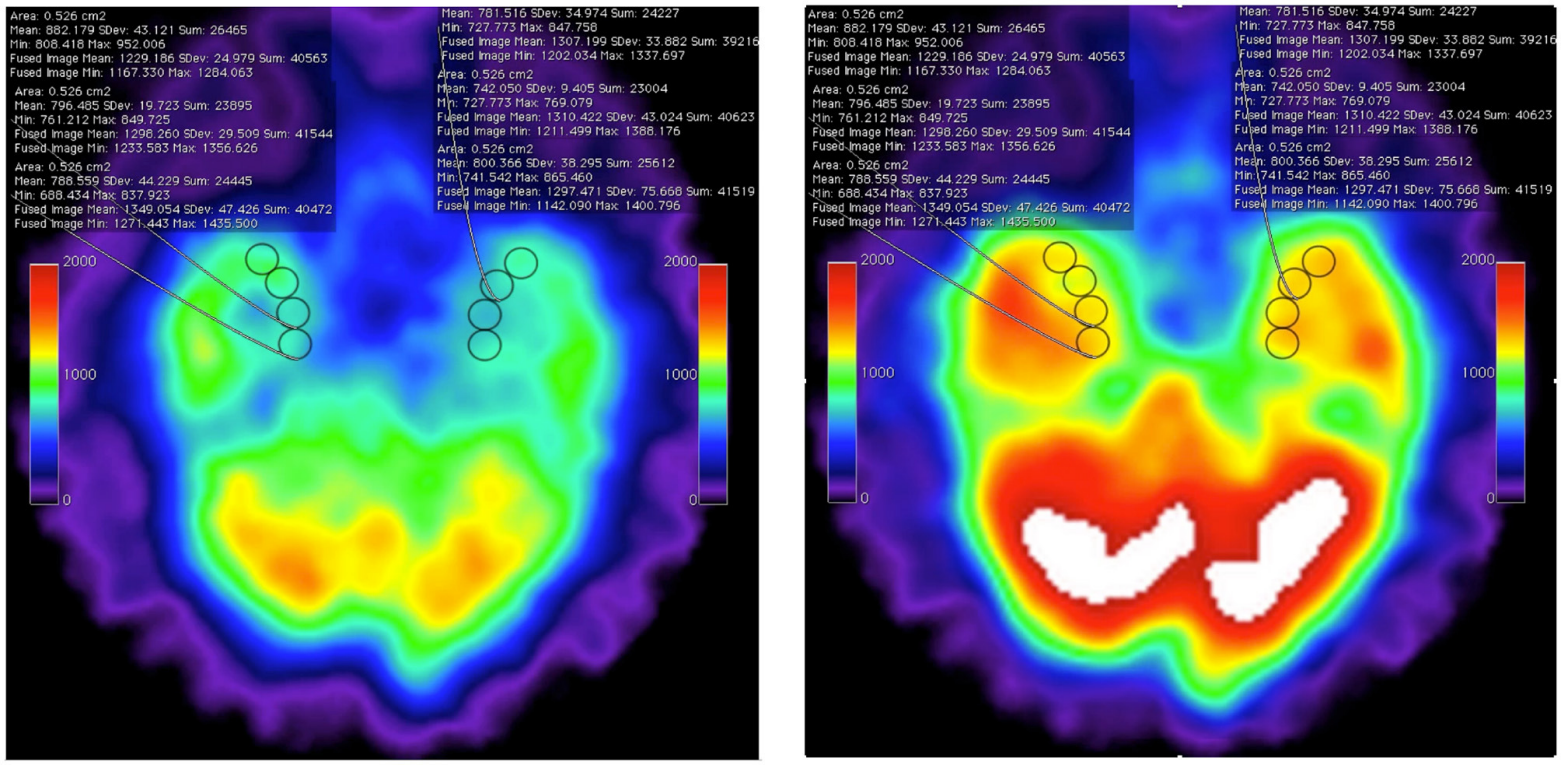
Axial SPECT imaging data from pre-HBOT baseline (left) and post 1-h HBOT data set (right) showing the inferior temporal lobes with eight regions of interest (ROI) in the medial temporal lobes. The statistical overlay documents increased intracellular GSH levels by Tc-99 HMPAO sequestration. These still images are captured from frame 1 and frame 20 of post-processed fusion of the two individual datasets performed on open-source Osirix clinical workstation software (see the source fusion video in Supplementary material). The “fused image” statistical data are obtained from the post-1-h HBOT HMPAO SPECT exam. Note that, at all ROIs, the uptake is increased over baseline by <30% [research data, Harch et al. (59)].
